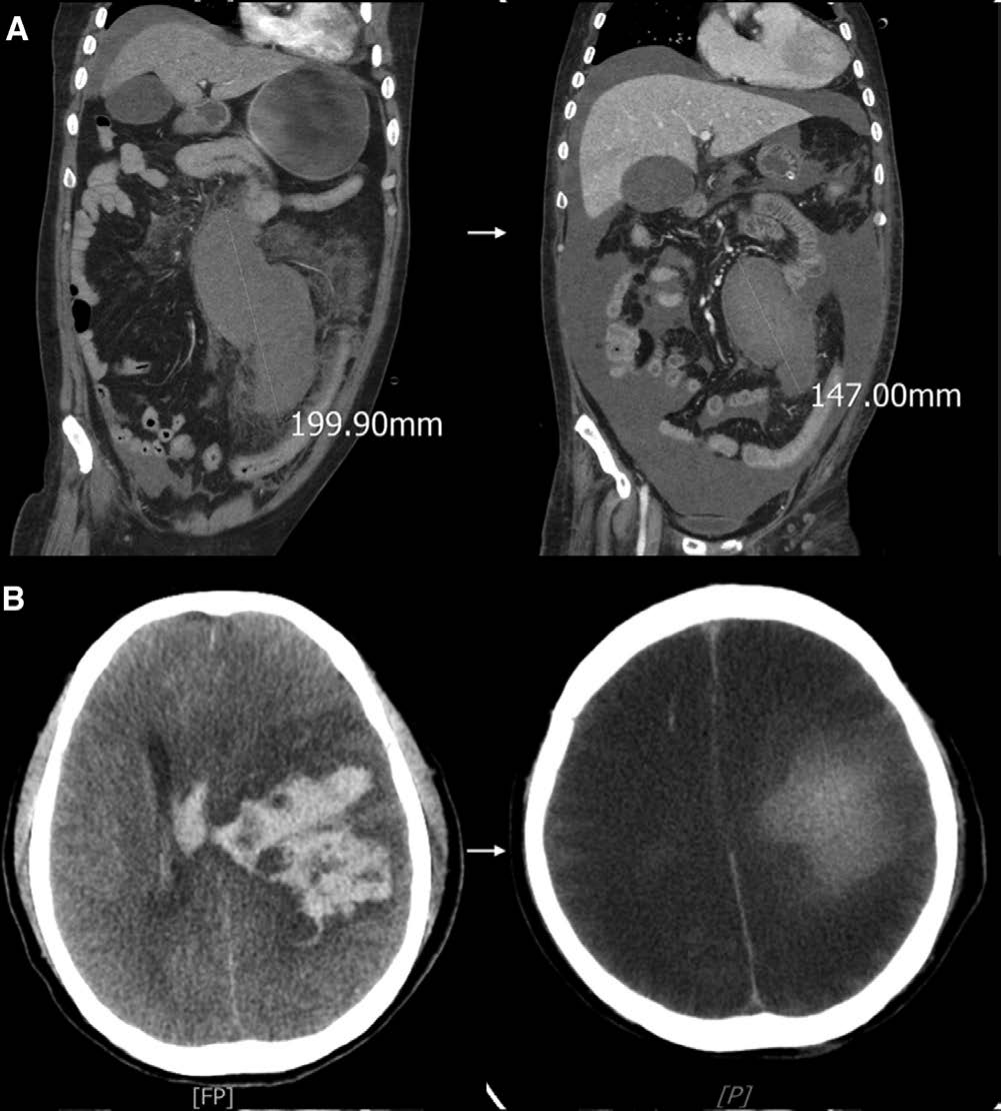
Bleeding before and after the treatment of acquired hemophilia. (A) A coronal section of the abdomen computed tomography showed the intraperitoneal hematoma spreading along the left psoas muscle (left). The size of the intraperitoneal hematoma decreased after treatment (right). (B) The transverse section of the brain computed tomography showed intracranial hemorrhage at the left basal ganglia and frontoparietal lobe with midline shifting to the right (left). The intracranial hemorrhage was improved after treatment (right).
